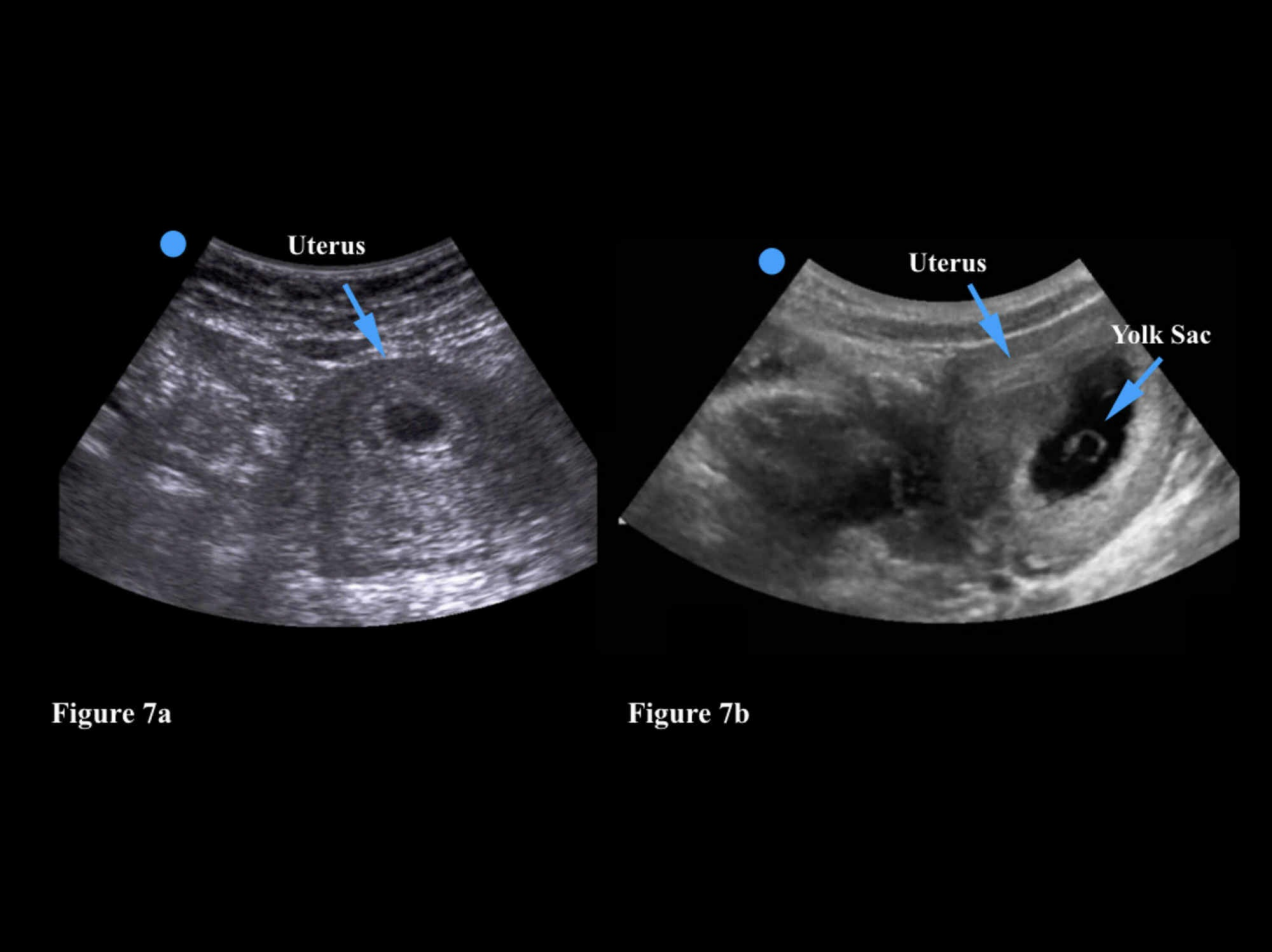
Figure 7a: An empty gestational sac within a uterus may signify a very early pregnancy but can also indicate an ectopic pregnancy. Figure 7b: This gestational sac has a circular yolk sac (often called the diamond ring sign) which is sonographic evidence of an intrauterine pregnancy.
